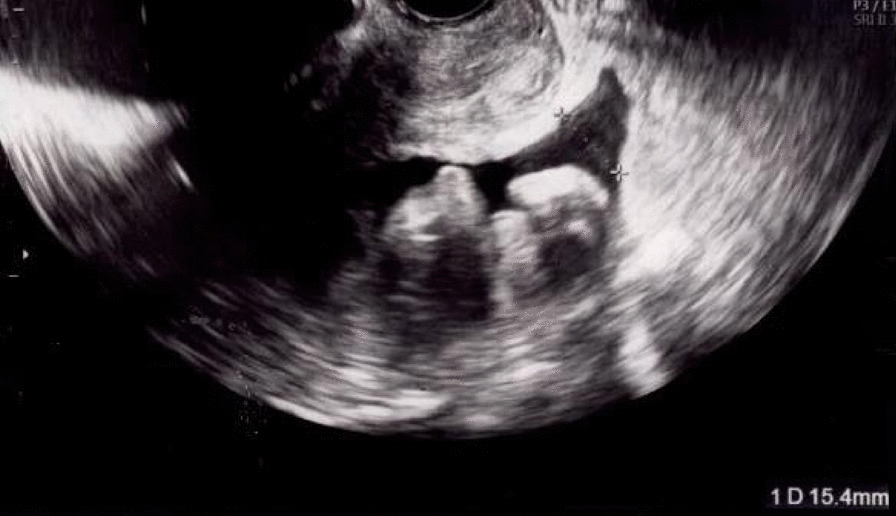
Ultrasonography showing mild ascites 2 days after oocyte retrieval
